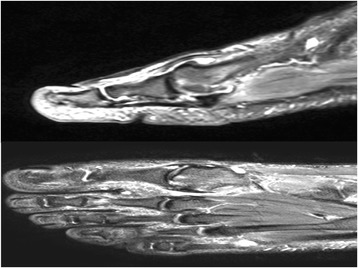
MRI of the 39-year-old man right foot, sagittal and axial view of the MTP joint
